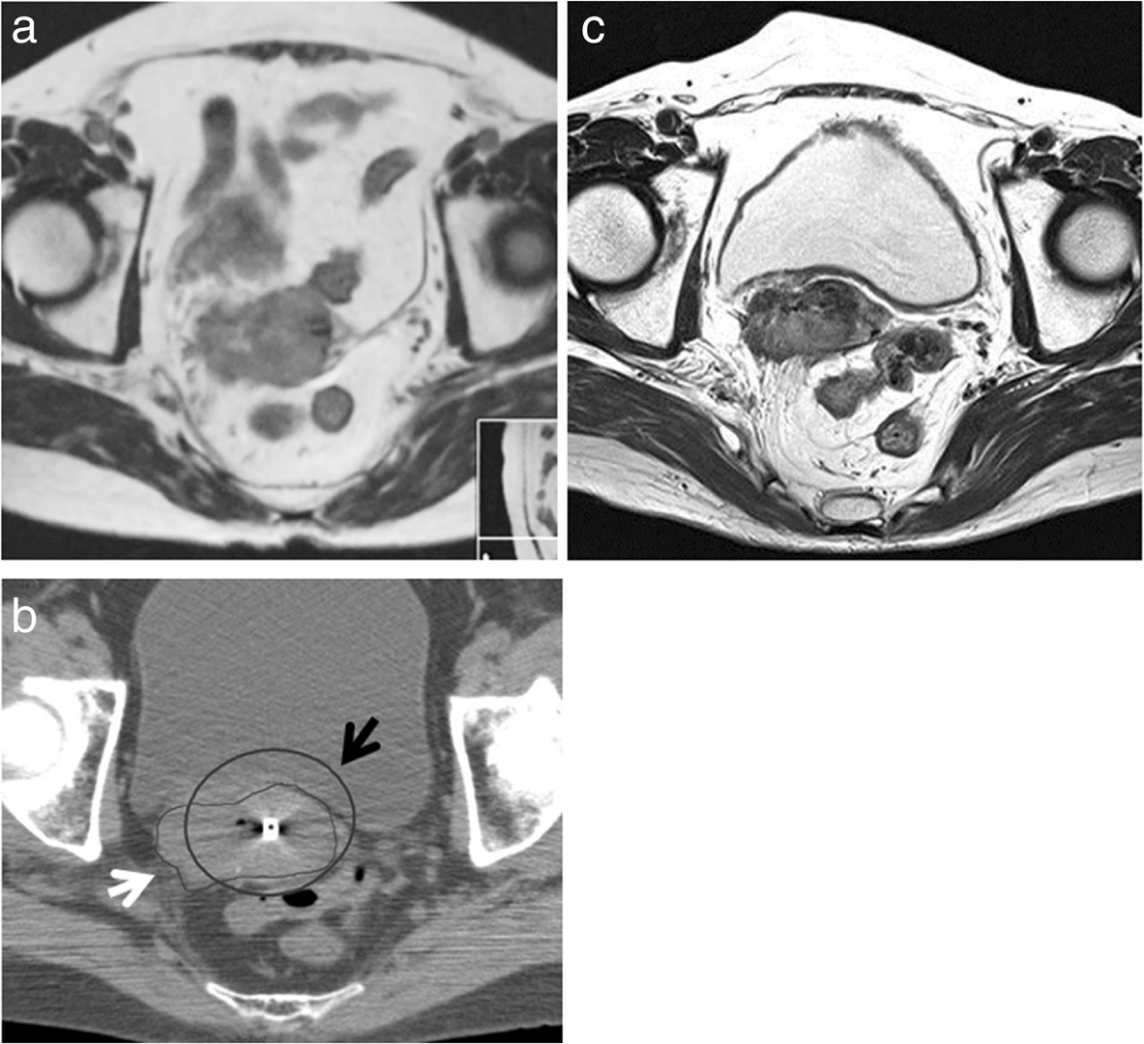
Representative images of patient who experienced local relapse. a. Axial MR T2 weighted image before treatment. Tumor extends to right pelvic wall. b. Axial CT image at the first session of intracavitary brachytherapy (ICBT). Tumor still extends to right pelvic wall after 40 Gy of whole pelvic EBRT. Black arrow represents isodose line of 60 Gy (EQD_2_) and white arrow HR-CTV at the time of brachytherapy. c. Axial MR T2 weighted image 3 months after completion of chemoradiotherapy. Persistent disease was found in right parametrium.
